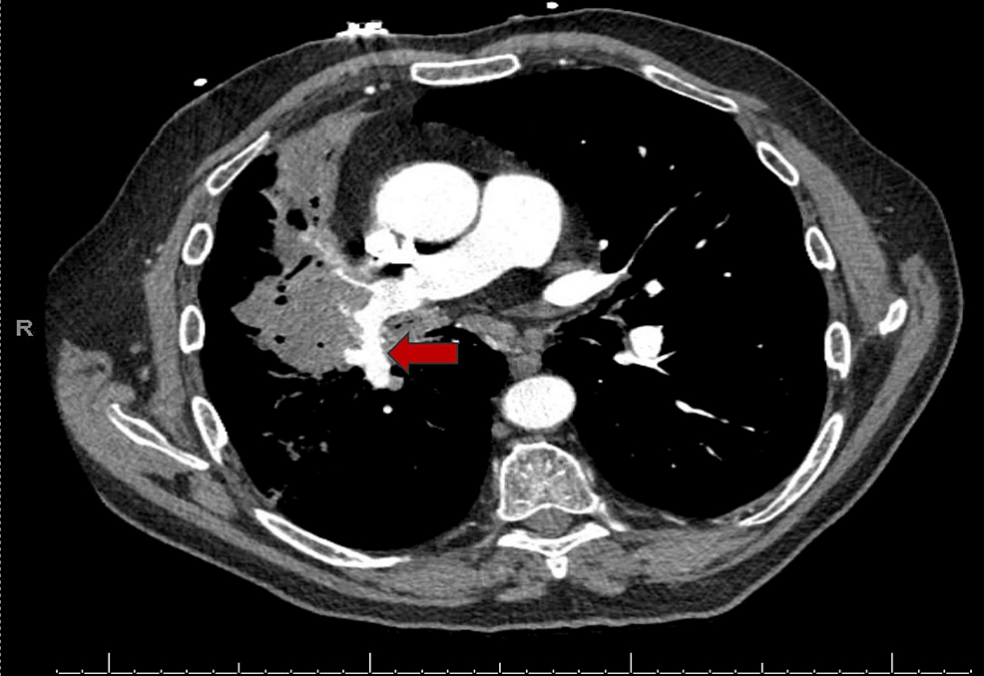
CT pulmonary angiogram demonstrating pseudoaneurysm of the right interlobar pulmonary artery (red arrow)
